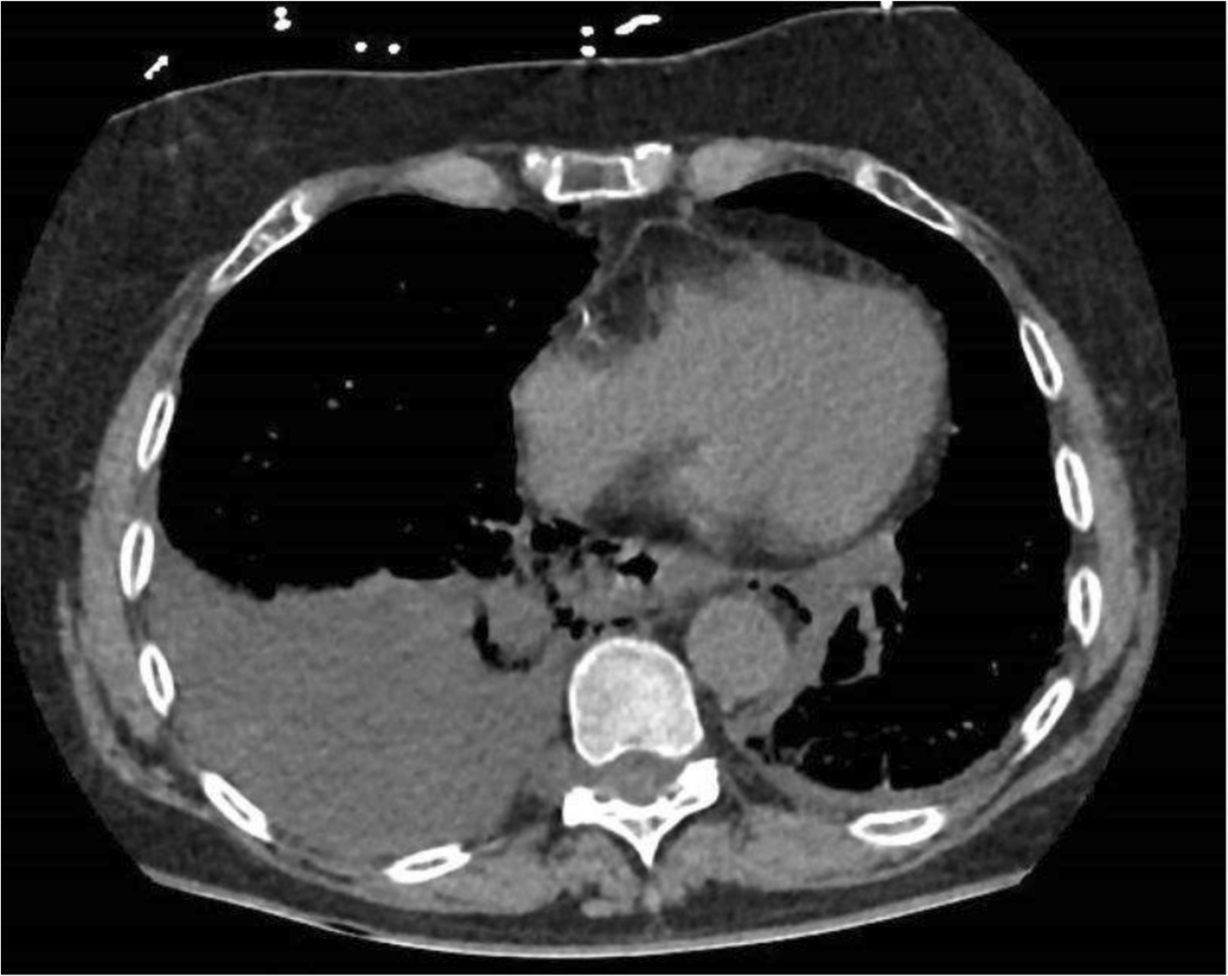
Axial CT showing a right pleural effusion, mediastinal air and esophageal wall disruption in a patient with spontaneous EP (Boerhaaves). Patient managed by right thoracotomy and laparotomy
